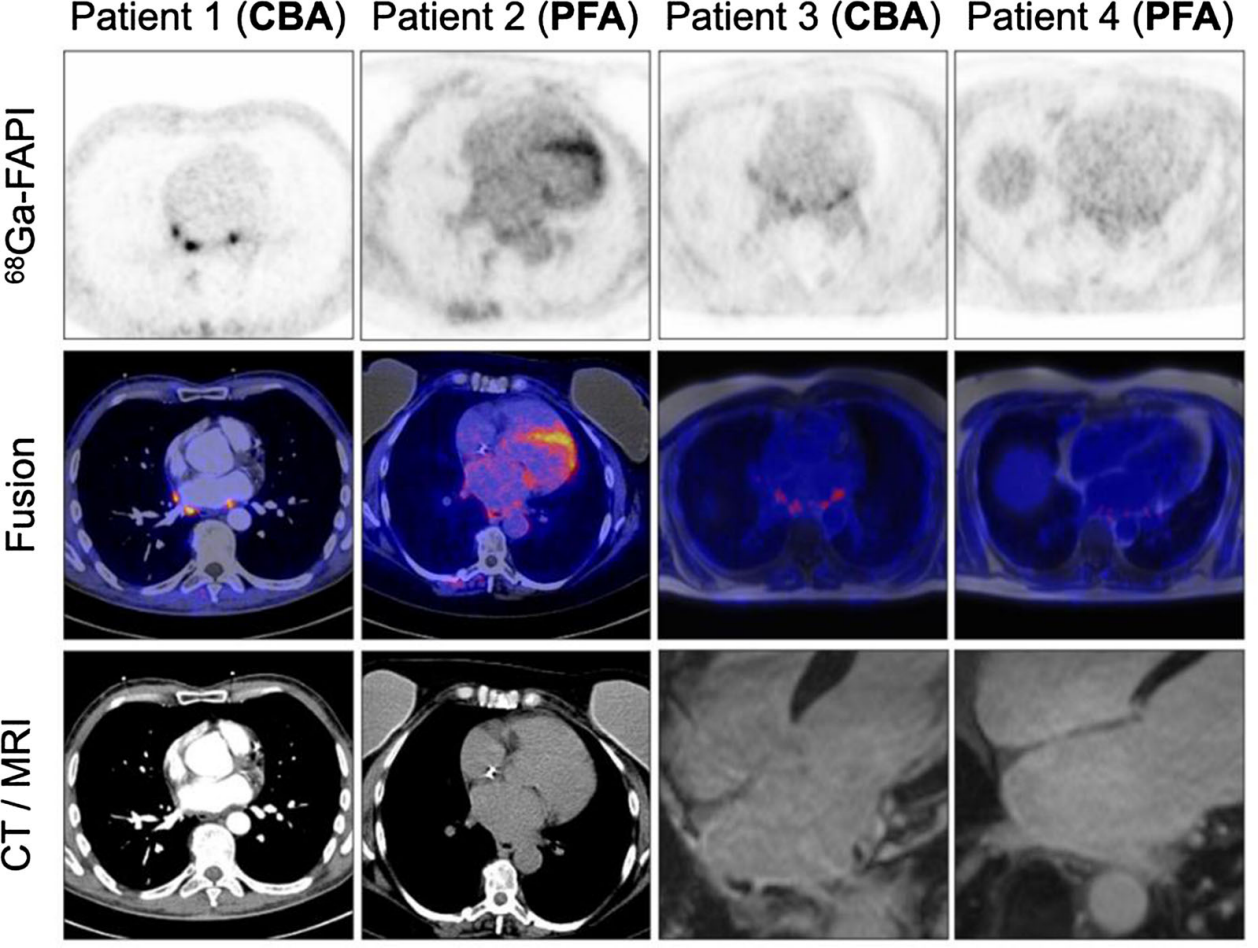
Patient 1 received CBA and showed intense tracer uptake in PVs; Patient 2 underwent PFA 7 days before imaging and showed mildly increased tracer uptake in the ostia of PVs. Of note, this patient had persistent AF, as well as severely dilated left atrium (left atrial volume of 40 mL·cm^−2^) which might have contributed to FAPI uptake as well. Incidental diffuse uptake in the left ventricle may possibly reflect myocardial remodeling in the setting of DCM; Patient 3 having undergone CBA and PET/MRI revealed moderate tracer uptake with corresponding late gadolinium enhancement (LGE) of the left atrium; Patient 4: received PFA and no visual tracer uptake can be observed in PET nor LGE in MRI. *CBA*, cryoballoon ablation; *PVs*, pulmonary veins; *PFA*, pulse field ablation; *PET*, positron emission tomography; *CT*, computer tomography; *MRI*, magnetic resonance imaging
